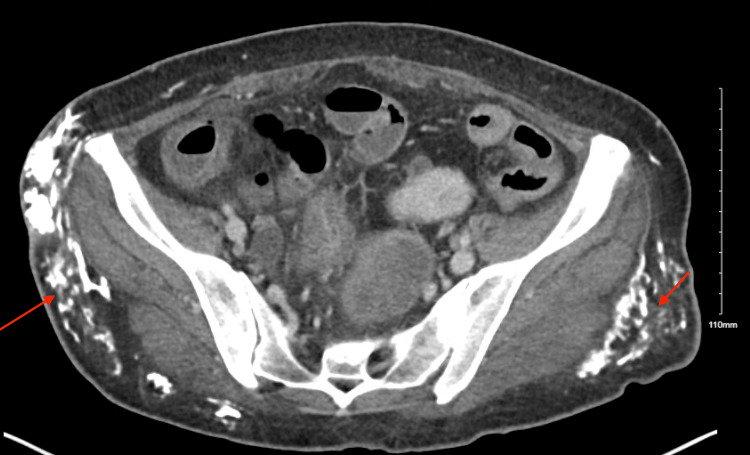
Axial computed tomography image of the abdomen and pelvis demonstrating extensive nodular calcifications within the subcutaneous tissues of the bilateral gluteal and lateral pelvic soft tissues (arrows), consistent with extensive soft tissue calcinosis
